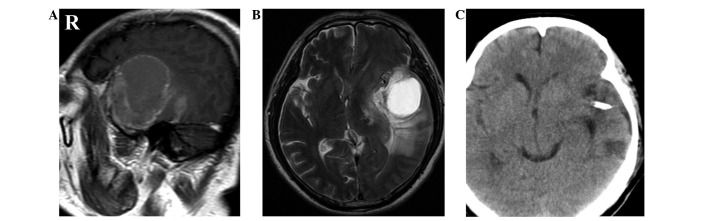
(A) T_1_-weighted magnetic resonance imaging (MRI) showing an enhanced ring-shaped mass around the cyst cavity within the left temporal region. (B) T_2_-weighted MRI showing a cyst cavity with surrounding edema. (C) Computed tomography (CT) showing cyst fluid resorption following puncture drainage.
